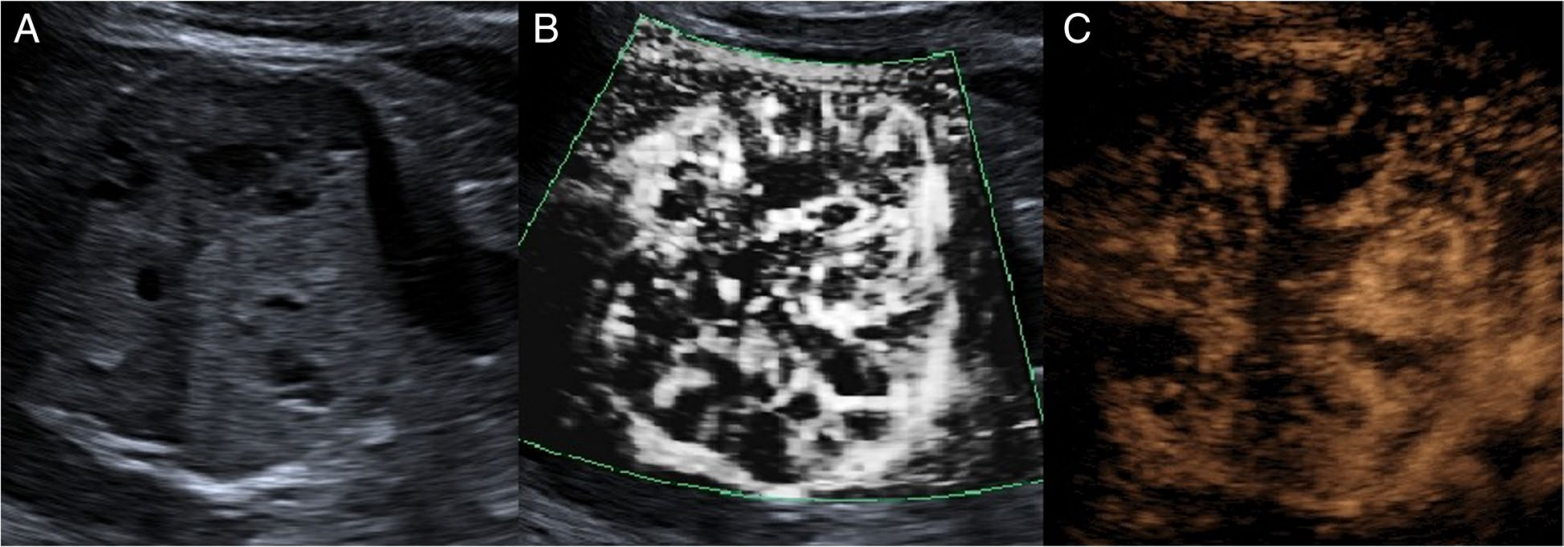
A solid tumor of the right kidney A ultrasound imaging, isoechoic mass with unclear boundary, regular shape, and inhomogeneous internal echo, with hypoecho and echoless area and small fluid area B SMI the growth from the periphery to the interior was tortuous and disorderly, with multiple branching vessels, and the vascular architecture was type V C MFI images showed the tortuous and disorderly growth of multi-branched vessels from the periphery to the interior. The vascular architecture was type V. Pathological results showed clear cell renal cell carcinoma
